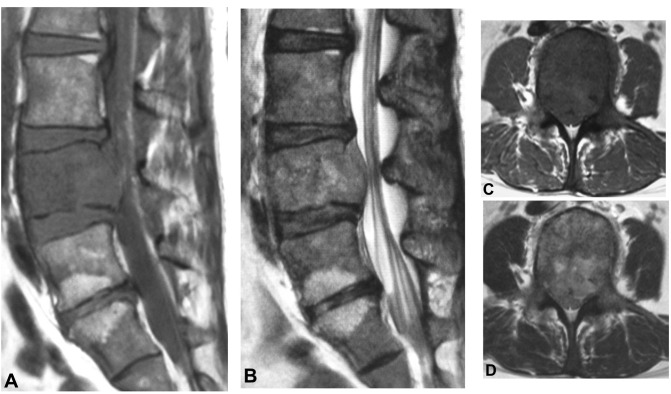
MRI of the lumbar spine. (A and C) T1- and (B and D) T2-weighted MRI of the lumbar spine in the (A and B) sagittal and (C and D) axial planes. The MRI shows L4 vertebral collapse and an extradural mass compressing the dura mater. The lesion extending from the L4 vertebral body to bilateral pedicles shows intermediate signal intensity on T1-weighted images and heterogeneous with mixed high and intermediate signal intensities on T2-weighted images. MRI, magnetic resonance imaging.
